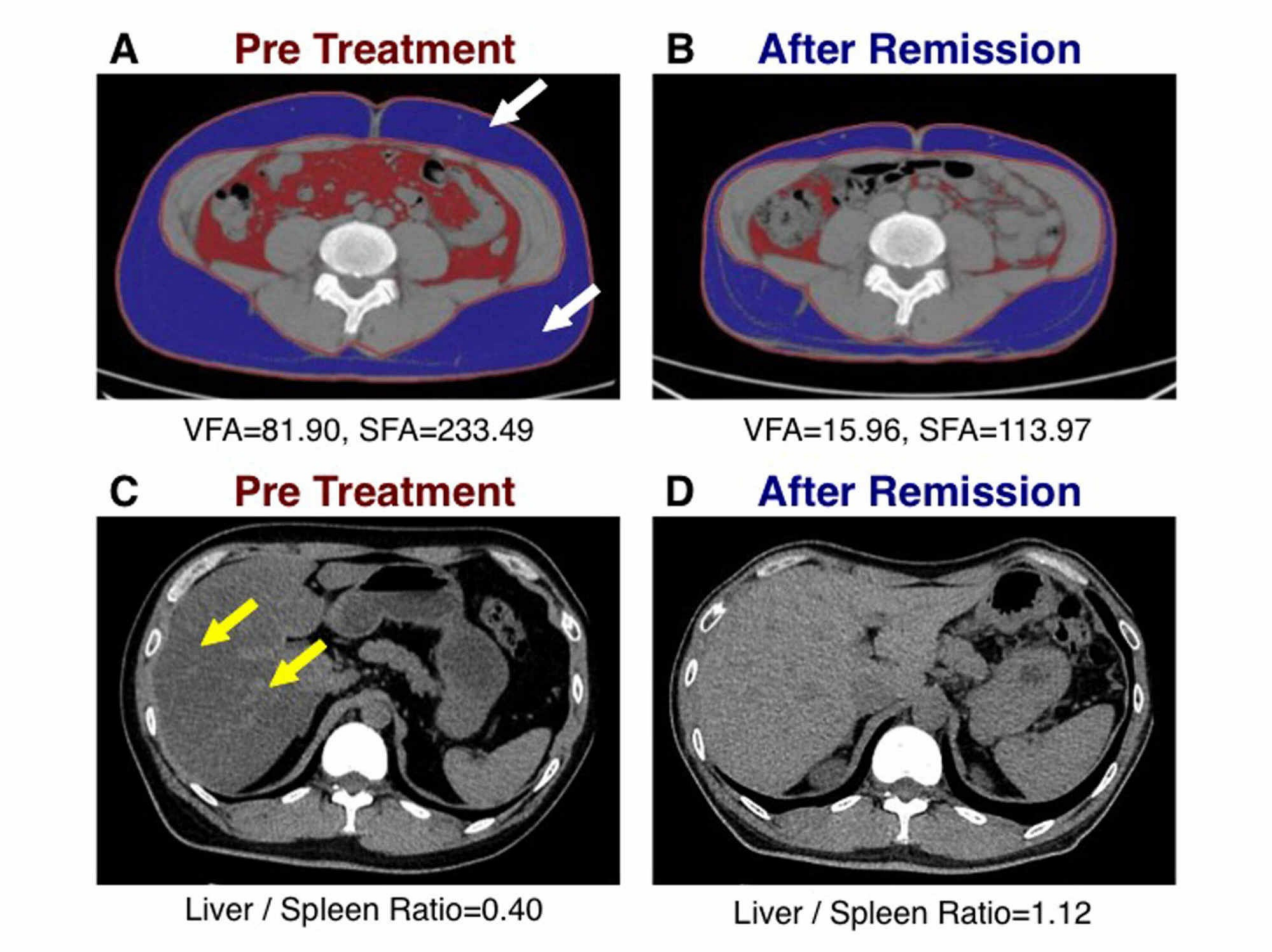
Changes in abdominal and liver fat accumulation after remission of diabetes Fat scan images and abdominal computed tomography images before therapy and after remission of diabetes. Blue areas indicated subcutaneous fat and red areas indicated visceral fat assessed by fat scan; VFA: visceral fat area (cm^2^), SFA: subcutaneous fat area (cm^2^). White arrows indicate the abdominal subcutaneous fat accumulation and yellow arrows indicate fatty liver. A: Fat scan image before therapy; B: Fat scan image after remission; C: Liver and spleen image before therapy; D: Liver and spleen image after remission
